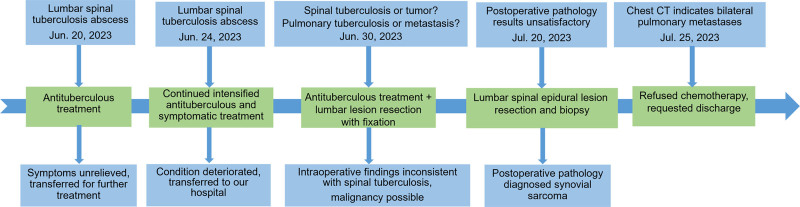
The timeline of the diagnosis and treatment course of the patient in the present case. CT = computed tomography.
